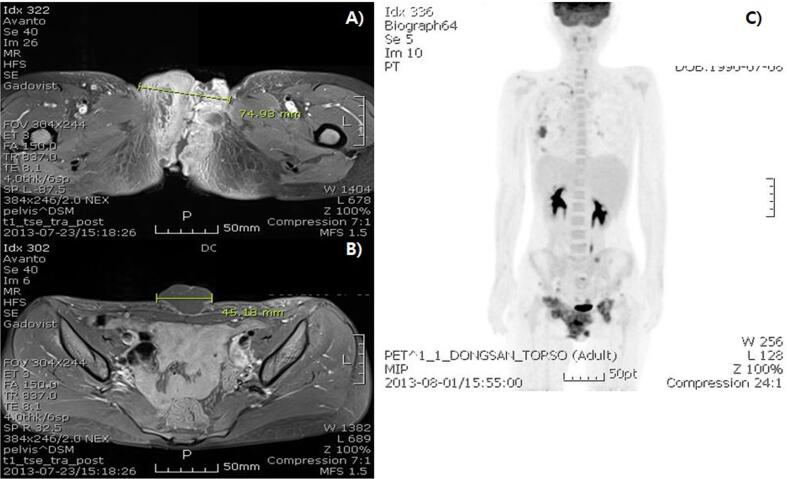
(a) MRI shows large, irregular shaped, infiltrating appearing mass with multifocal intratumoral necrosis at both sides of vulva and perineal area, mainly located at subcutaneous layer. (b) MRI also shows subcutaneous enhancing nodules at lower anterior abdominal wall. (c) PET CT shows distant metastases to lung, left pleura, bone and soft tissue and nodal metastases at left external iliac and right inguinal lesion.
